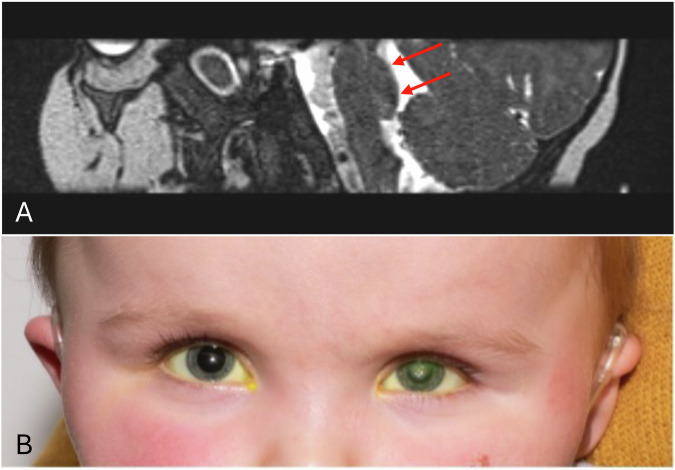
Pontine tegmental cap dysplasia. A Pons sagittal T2 weighted magnetic resonance image: ventral hypoplasia and appearance of a rounded “cap” (red arrows) projecting into the 4th ventricle. B Two-year old with bilateral corneal anaesthesia and left corneal opacity. Magnetic resonance imaging demonstrated pontine tegmental cap dysplasia but also associated absence of auditory and vestibular nerves.
